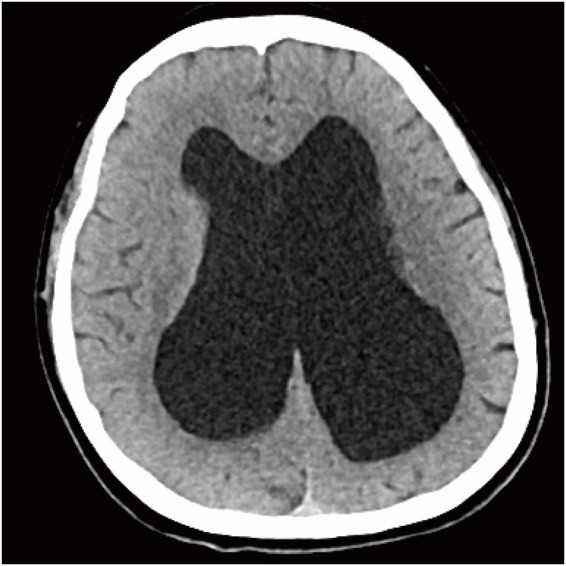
Representative axial head CT demonstrating hydrocephalus. Axial CT image (brain window) shows marked, symmetric dilatation of the bilateral lateral ventricles, with ballooning of the frontal horns and prominent enlargement of the ventricular bodies/occipital horns, accompanied by thinning of the surrounding cerebral mantle, consistent with severe ventriculomegaly/hydrocephalus.
